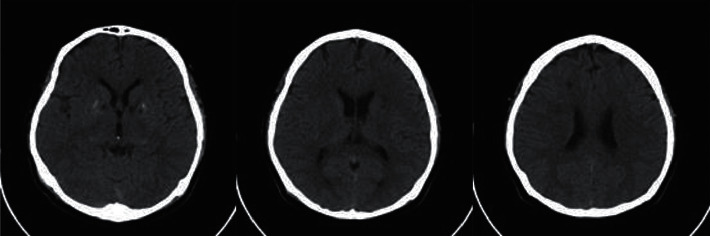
Multiple lacunar hypodense lesions on the CT scan.
